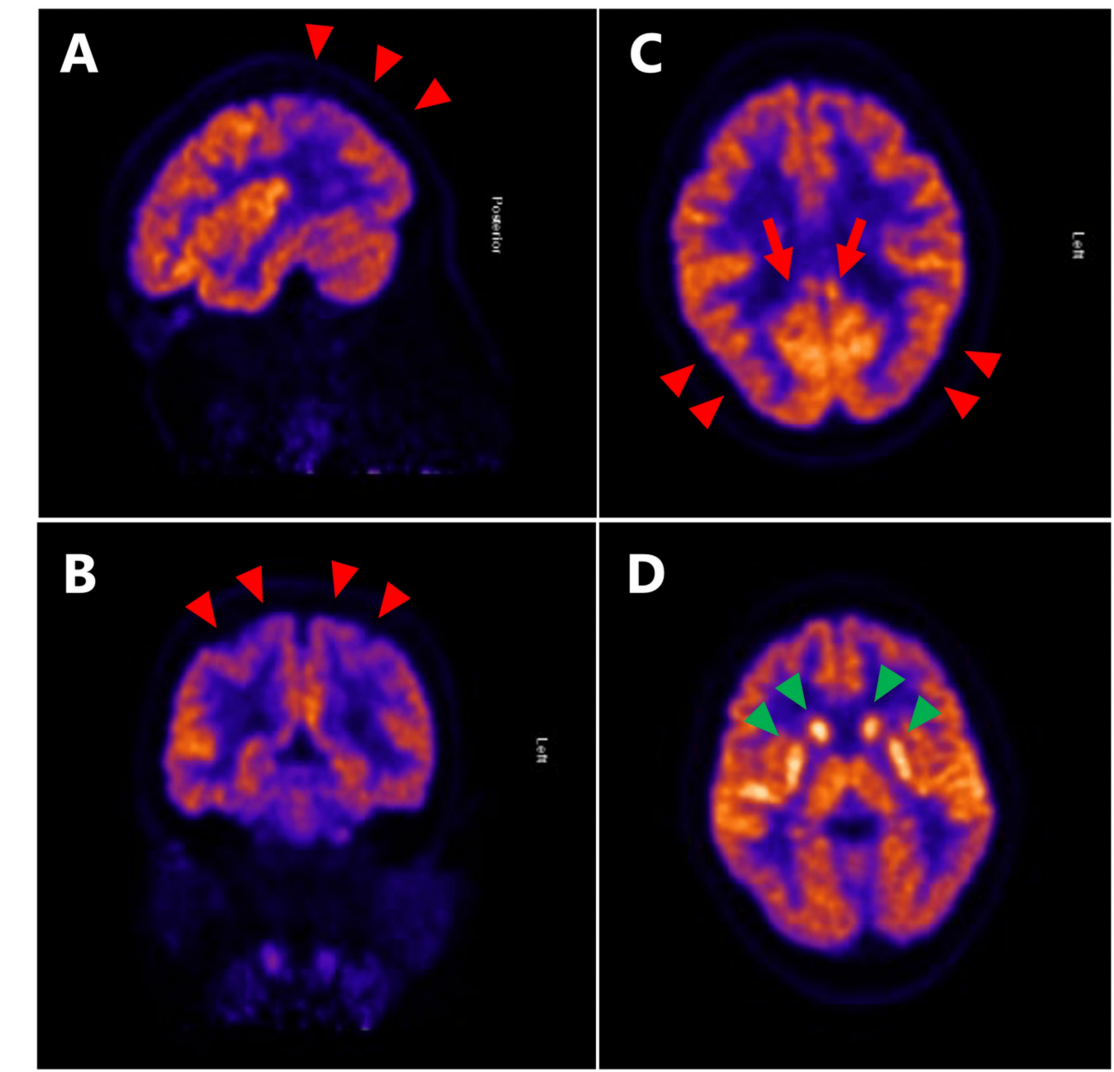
Fluorodeoxyglucose positron emission tomography (FDG-PET) findings of the brain. There is significantly reduced glucose metabolism in the parietal lobes (A, B, and C, red arrowheads), extending into the occipital lobes while sparing the visual cortex (C, red arrow). There is increased metabolism in the caudate, putamen, and thalamus (D, green arrowheads).
